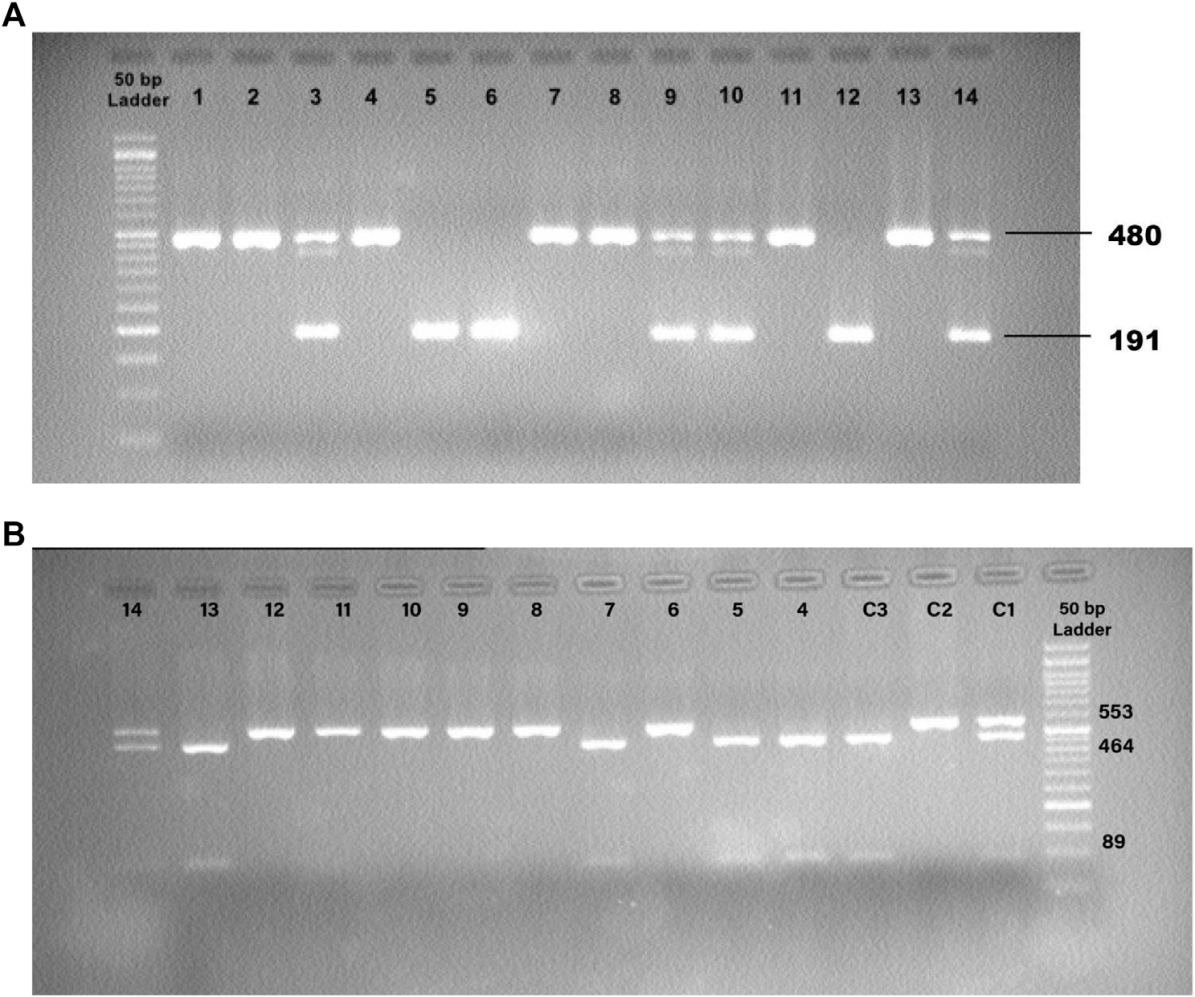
(A) Detection of the PCR Products for ACE1 Insertion/Deletion (I/D) Polymorphism. 12: Control, DD; 13: Control, II; 14: Control, ID; 1,2,4,7,8,11: II; 5,6: DD; 3,9,10: ID. (B) Detection of the PCR-RFLP Products for ACE2 rs1978124 SNP. C1: Control, CT; C2: Control, TT; C3: Control, CC; 4, 5, 7, 13: CC; 6, 8, 9, 10, 11, 12: TT; 14: CT.
